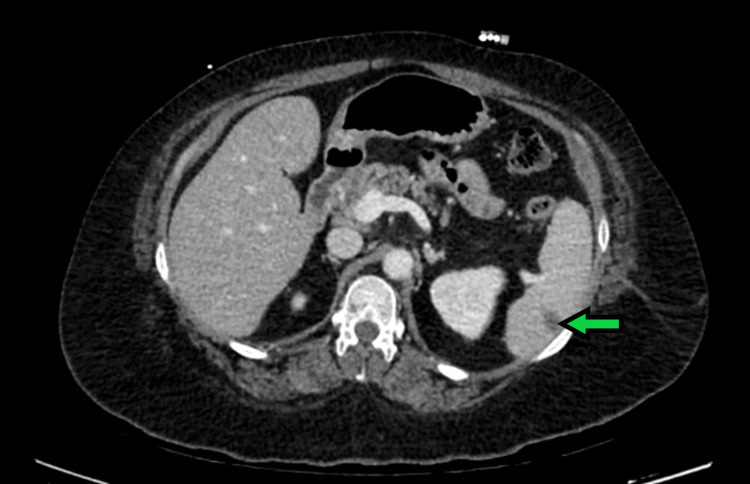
CT of the abdomen showing a wedge-shaped infarct of the spleen (arrow) CT: computed tomography
